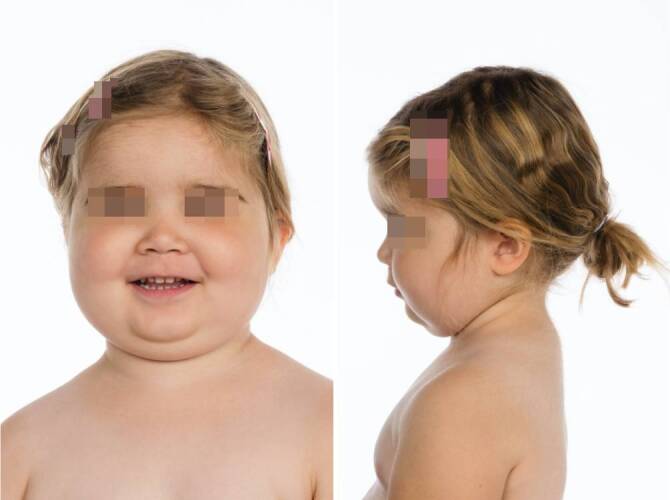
Laborchemisch zeigten sich eine Leukozytose (15.630/µl), Thrombozytose (416.000/µl) sowie eine Erhöhung des C‑reaktiven Proteins (10,2 mg/dl) als Ausdruck einer deutlichen Akute-Phase-Reaktion. Im Urin ließen sich eine Leukozyturie sowie kulturell *E. coli* nachweisen (Keimzahl 10^6^/ml). Die weitere infektiologische und immunologische Diagnostik blieb unauffällig, ebenso blieben Sonographien von Abdomen und Nieren ohne Hinweis auf eine Harntransportstörung.
